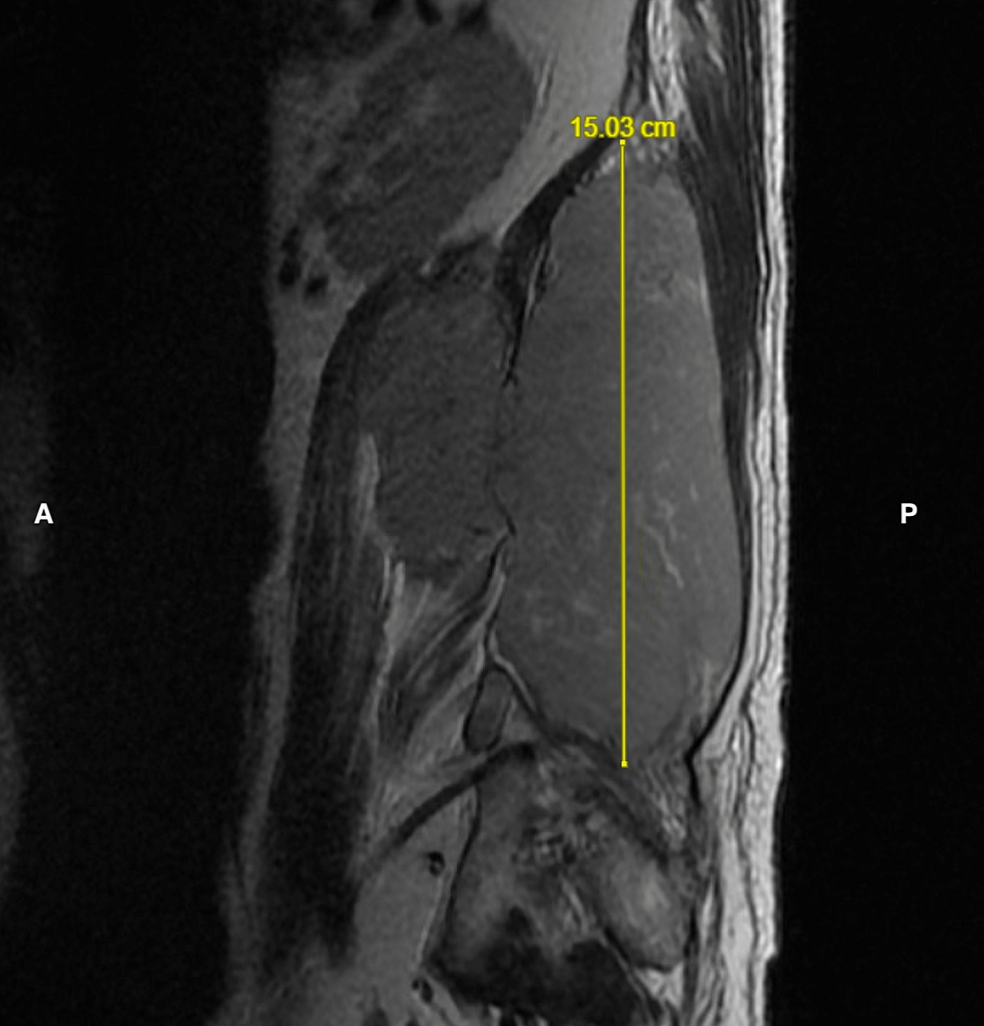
Soft tissue mass situated within the posterior side spanning approximately 15.03 cm craniocaudally in T2-weighted sagittal view. A = Anterior; P = Posterior
